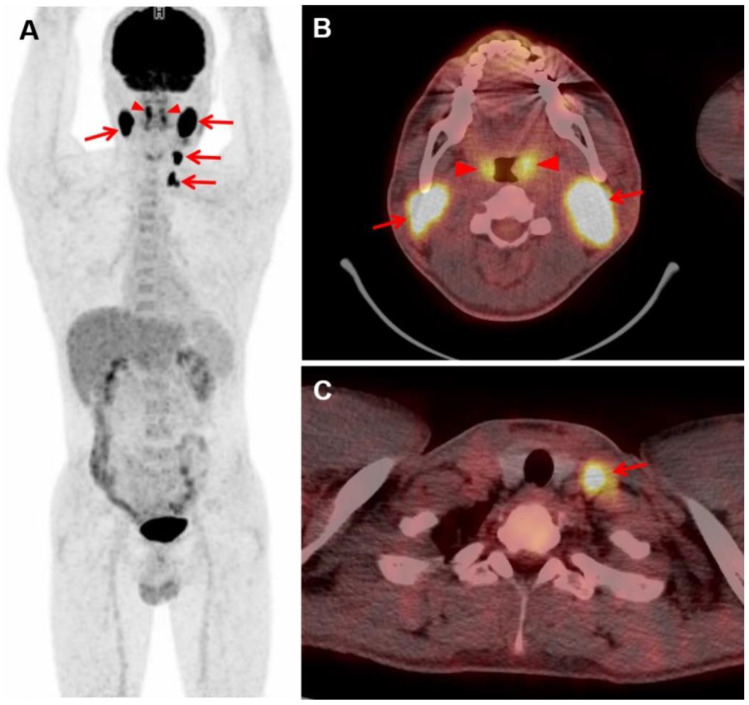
Baseline FDG PET/CT scan of a 36-year-old male patient with DLBCL confirmed by biopsy of the left tonsil. In maximum intensity projection PET (A) and transaxial fusion PET/CT (B,C) images, bilateral tonsils showed increased FDG uptake (arrowheads) and enlarged bilateral cervical lymph nodes showed intensely increased FDG uptake (arrows; SUVmax 23.2 in right cervical level II). The bone FDG uptake had intensity less than the liver and was considered BM PET-negative on visual analysis. However, he had BMBx-positive result. In radiomic analysis, two GLZLM-derived indices obtained from iliac crests were relatively high (HGZE 359.3 and SZHGE 229.0).
